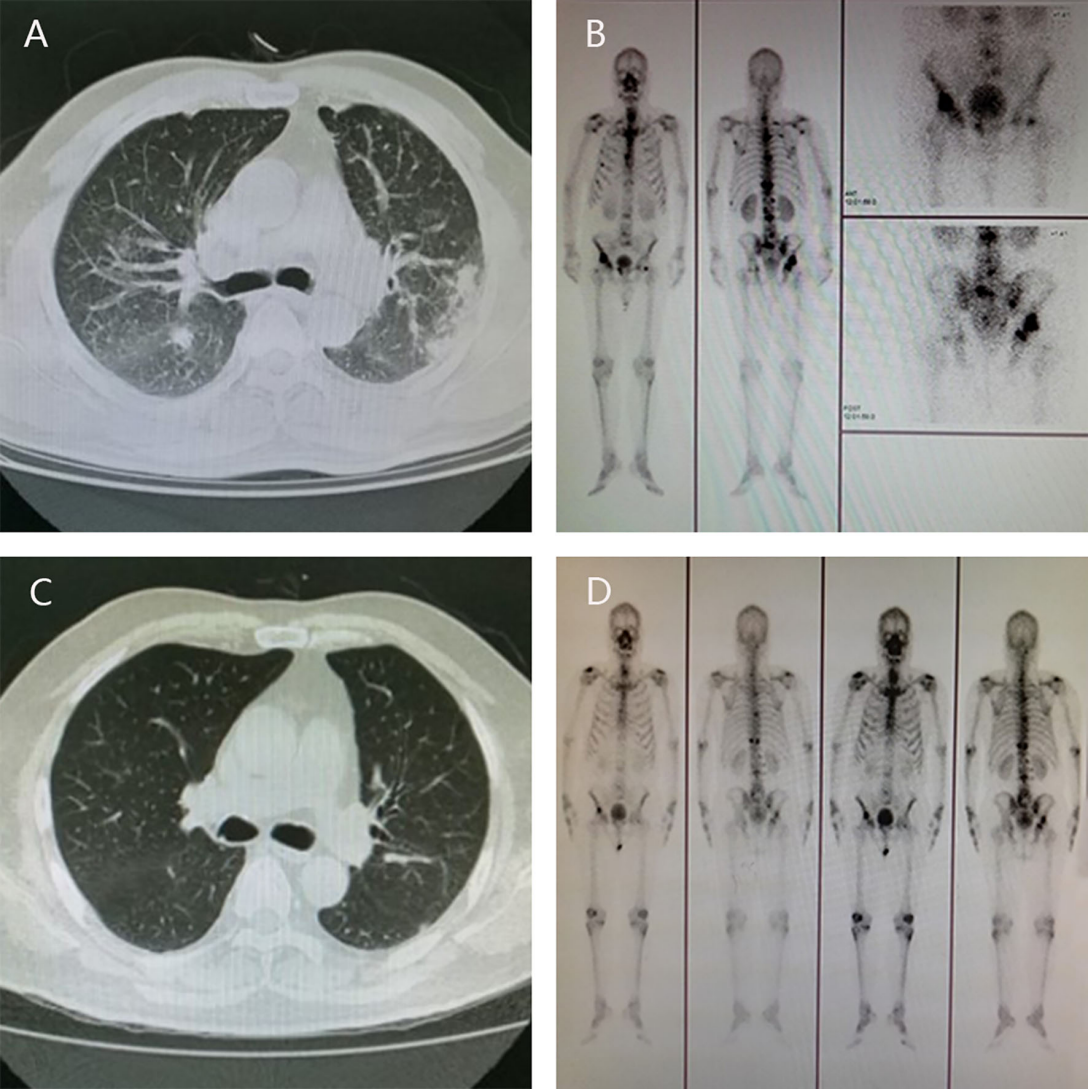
Radiographic change pre- and post-therapy. The results of CT scan showed metastatic tumors in bilateral lungs at diagnosis (A) and no visible metastatic tumors in the lungs after androgen deprivation therapy and six cycles of chemotherapy (C); Bone scan showed multiple metastases in the scapulae, ribs, sacroiliac joints, hip joints, thoracic vertebrae, lumbar vertebrae, etc at diagnosis (B) and the metastatic tumors in the bones lessened significantly (D).
